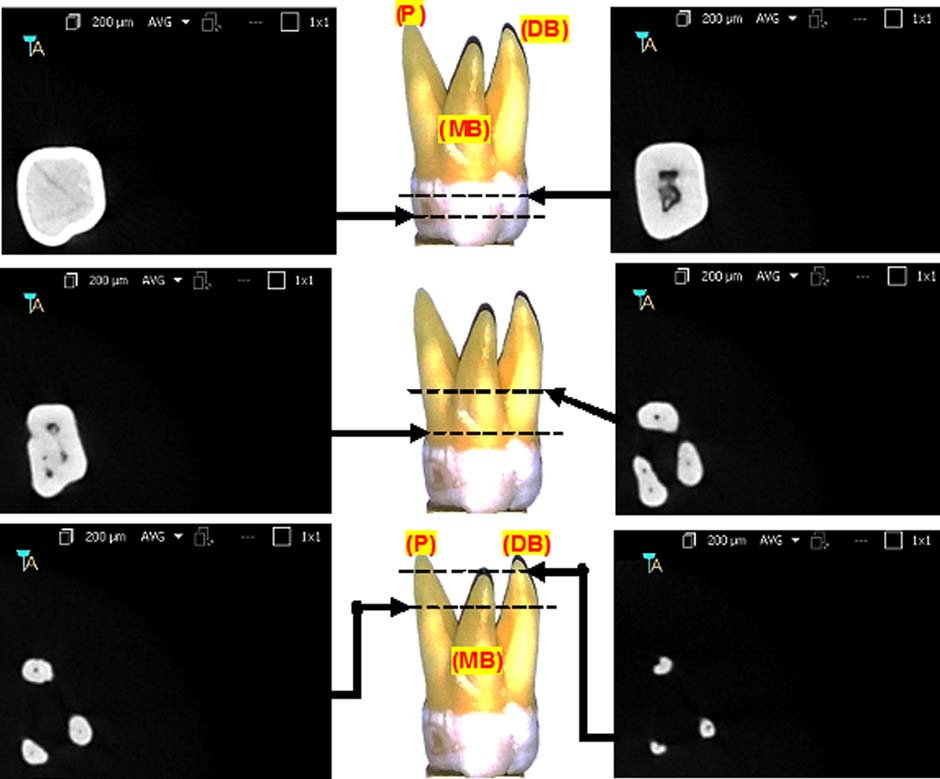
Internal anatomy of a typical maxillary 1^st^ molar (three roots) using CBCT at various anatomical levels.
